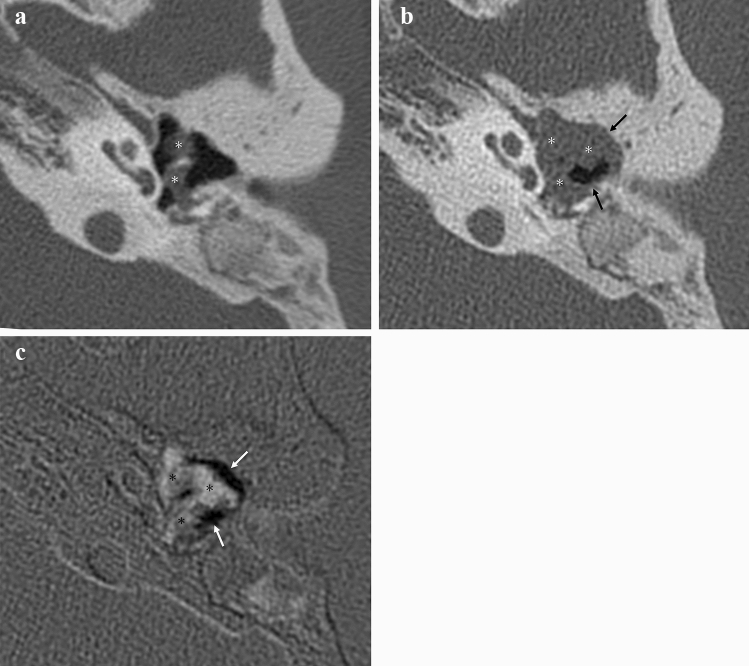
Postoperative recurrence of left pars flaccida cholesteatoma with relatively significant bone erosive changes. Postoperative temporal bone CT (a) about 9 years ago shows a soft tissue density (*) in the left tympanic and mastoid cavity. Temporal bone CT (b) before surgery for recurrence/residual shows increased soft tissue density (*) and adjacent progressive bony erosive changes (black arrows). In TSCT (c), progressive bone erosive changes are visualized as distinctive black areas (white arrows), and areas of increasing soft tissue density are visualized as white areas (*). TSCT, temporal subtraction CT
